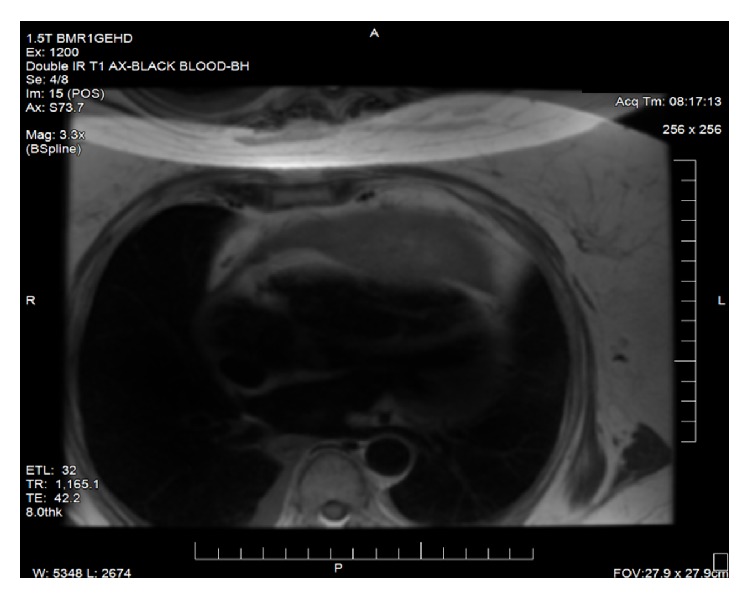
Cardiac MRI showing cystic solid mass arising in the pericardium.
